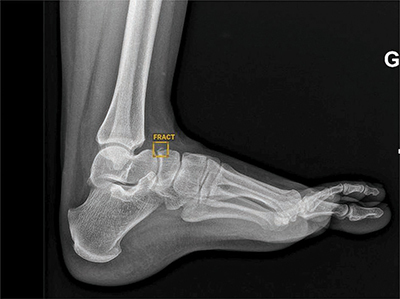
AI true positive response for fracture of navicular bone confirmed by the radiologist in charge of radiographic exams.
